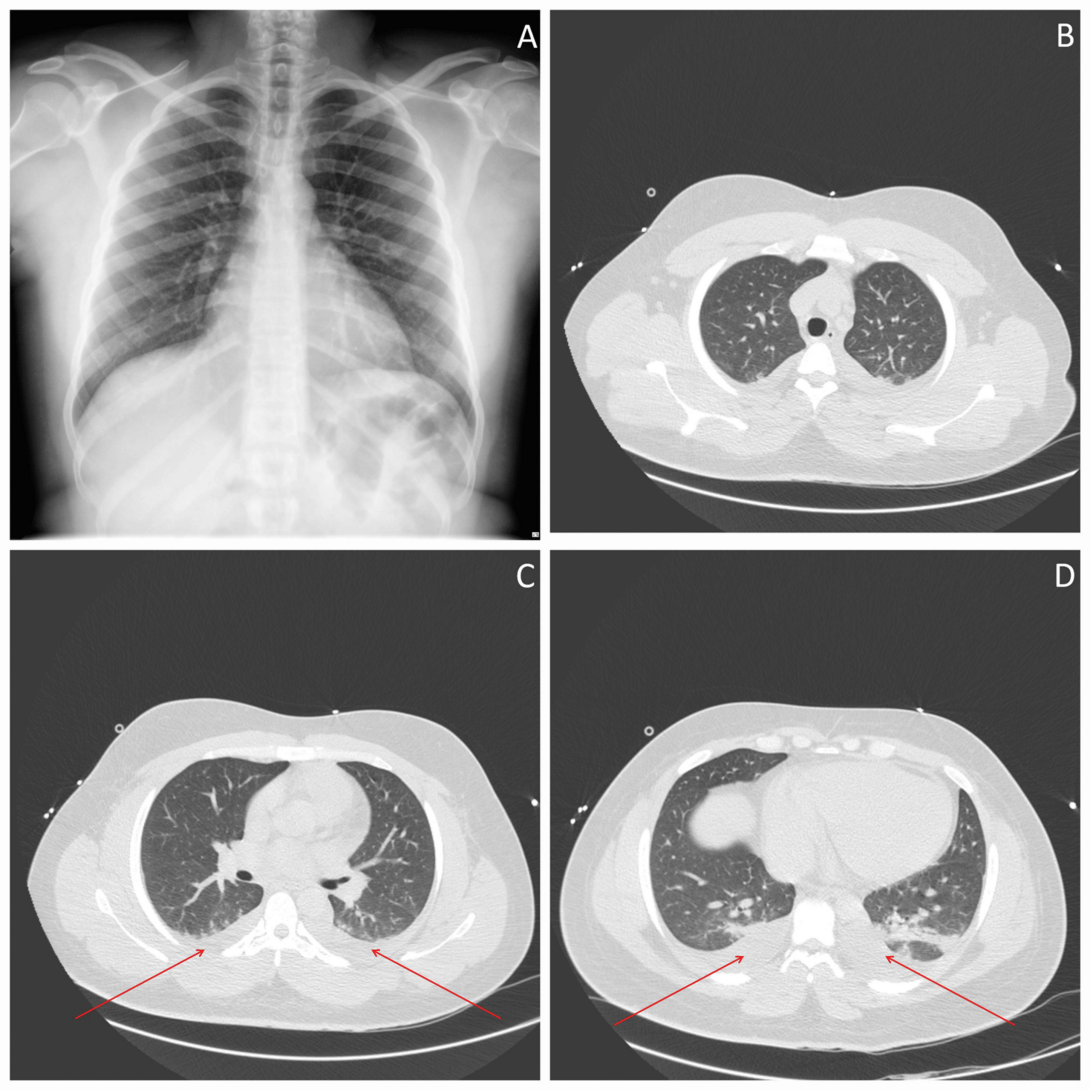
Thoracic imaging findings (A) Posteroanterior chest X-ray demonstrating clear lung fields with no obvious consolidations or infiltrates. (B-D) Sequential axial slices of chest CT from the upper to lower thorax. The scans confirm the complete absence of parenchymal pneumonia, infiltrates, or abscesses. Panel D reveals trace bilateral pleural effusions (posterior dependent densities) and generalized diffuse subcutaneous edema, consistent with the systemic presentation of Pontiac fever. CT: computed tomography
